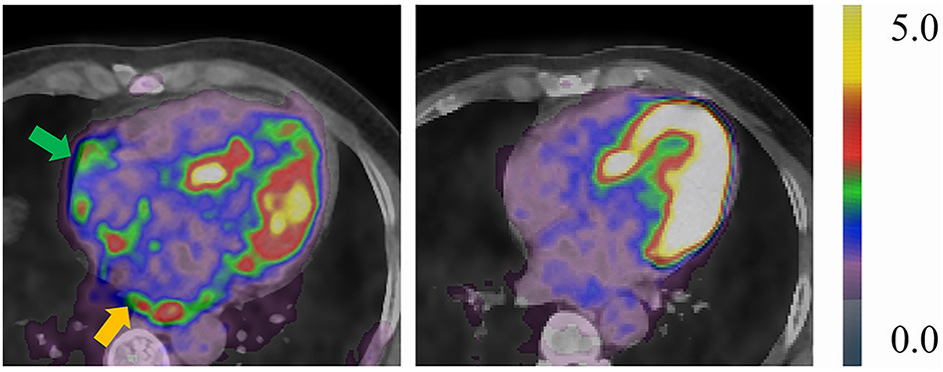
Representative case of a 49-year-old male PsAF patient before (left: TBR_LA_ = 2.16, TBR_RA_ = 1.79) and after RFCA (right: TBR_LA_ = 0.69, TBR_RA_ = 1.36). Yellow arrow indicates abnormal LA FDG uptake, green arrow indicates abnormal RA FDG uptake. Both LA and RA FDG activities reduced compared to baseline scans.
